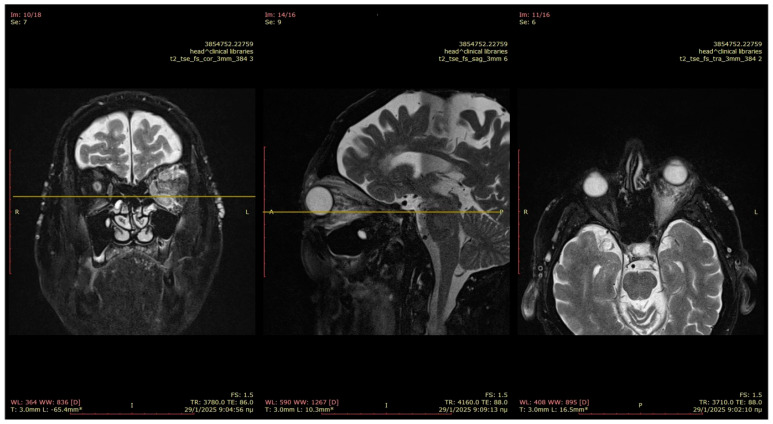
MRI, T2-weighted images from coronal, sagittal, and axial perspectives. Generalized thickening and edema of all the extraocular muscles, mostly evident in the lower rectus muscle.
